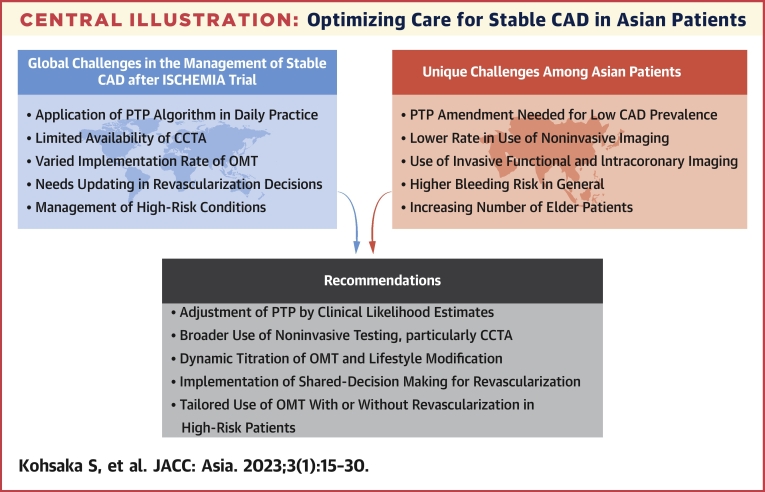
Optimizing Care for Stable CAD in Asian Patients With advances in medical therapy and revascularization techniques, outcomes in patients with stable coronary artery disease (CAD) have progressively improved. However, unmet needs still exist between guideline recommendations and pretest probability (PTP) estimation, application of noninvasive testing, pharmacotherapy, and revascularization in Asian countries. Updated decision making and management in patients with stable CAD, including renewed evidence, should be encouraged. CCTA = coronary computed tomographic angiography; ISCHEMIA = International Study of Comparative Health Effectiveness With Medical and Invasive Approaches; OMT = optimal medical therapy.
